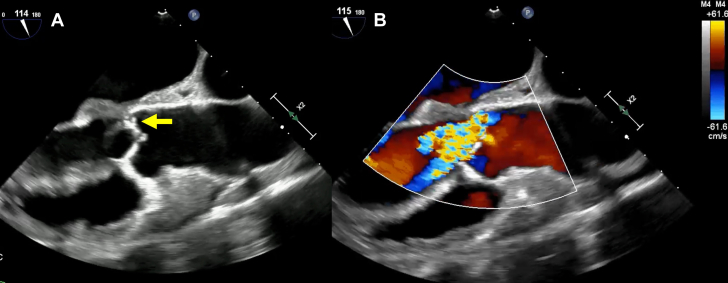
TEE and Color Doppler Imaging of the AV TEE (A) and color Doppler images (B), penetration into the NCC (yellow arrow), and severe aortic regurgitation originating from the NCC and RCC of the AV. AV = aortic valve; NCC = noncoronary cusp; RCC = right coronary cusp; TEE = transesophageal echocardiogram.
